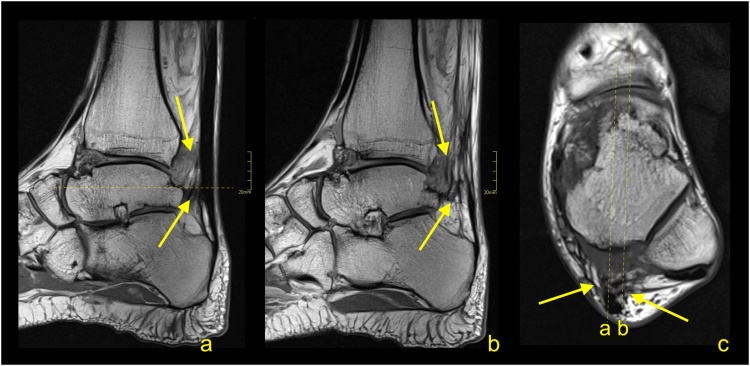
Status after elongation of the Achilles tendon in childhood. Clinical stiffness and reduced mobility in the ankle joint. MRI (a–c) revealed the extensive postoperative changes between the Achilles tendon and Kager's fat pad (straight arrow). A, b and c- PD- weighted.
